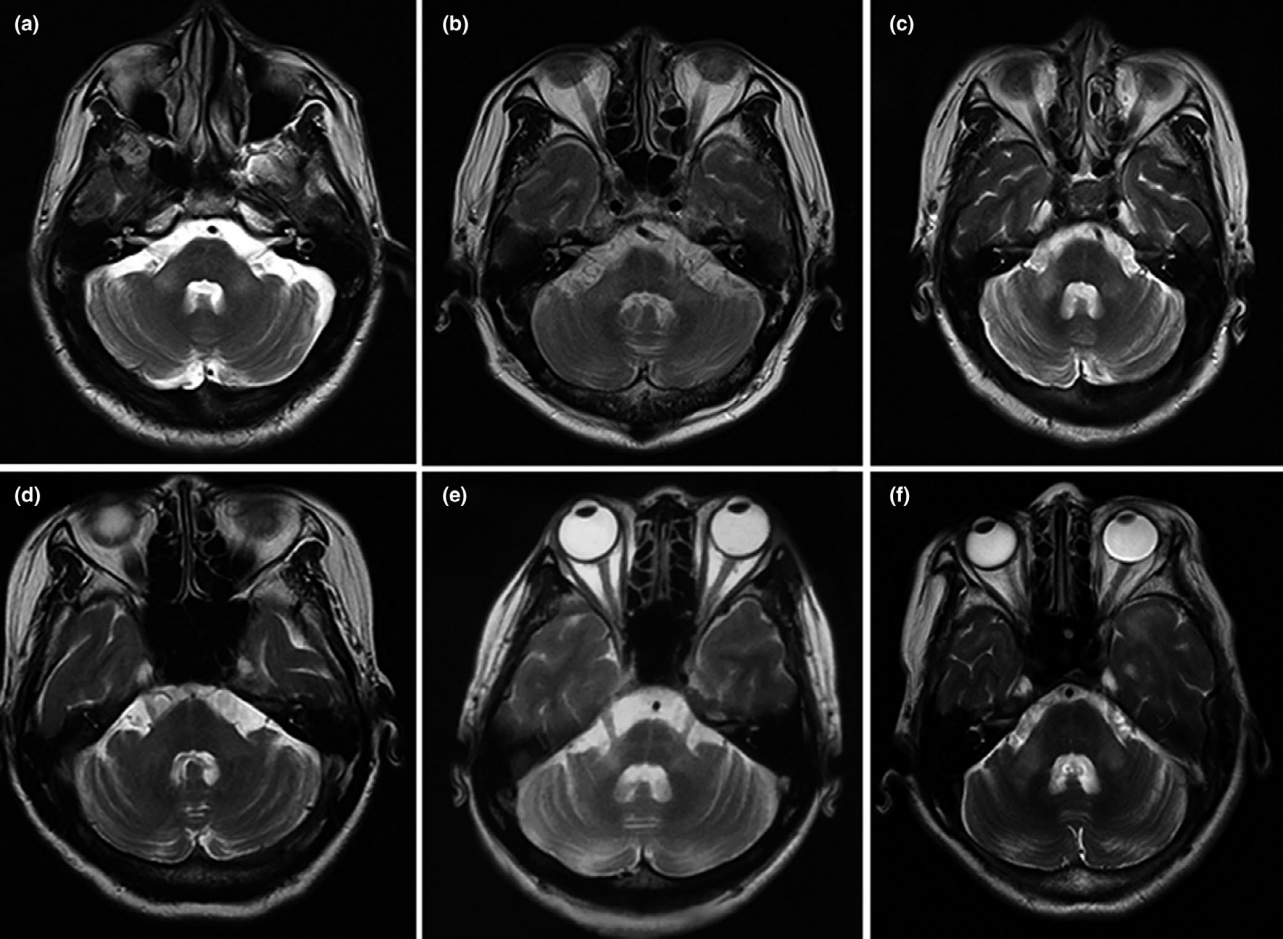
Brain MRI of six patients with multiple system atrophy. All patients show an increasing signal of both MCPs on T2‐weighted imaging. Axial pontine T2‐weighted imaging shows a clear vertical line and a fuzzy horizontal line of hyperintensity (a,c,d,f), and both clear horizontal and vertical lines of hyperintensity (“hot cross bun” sign) with mild atrophy of the brainstem, MCPs, and cerebellum (b,e)
